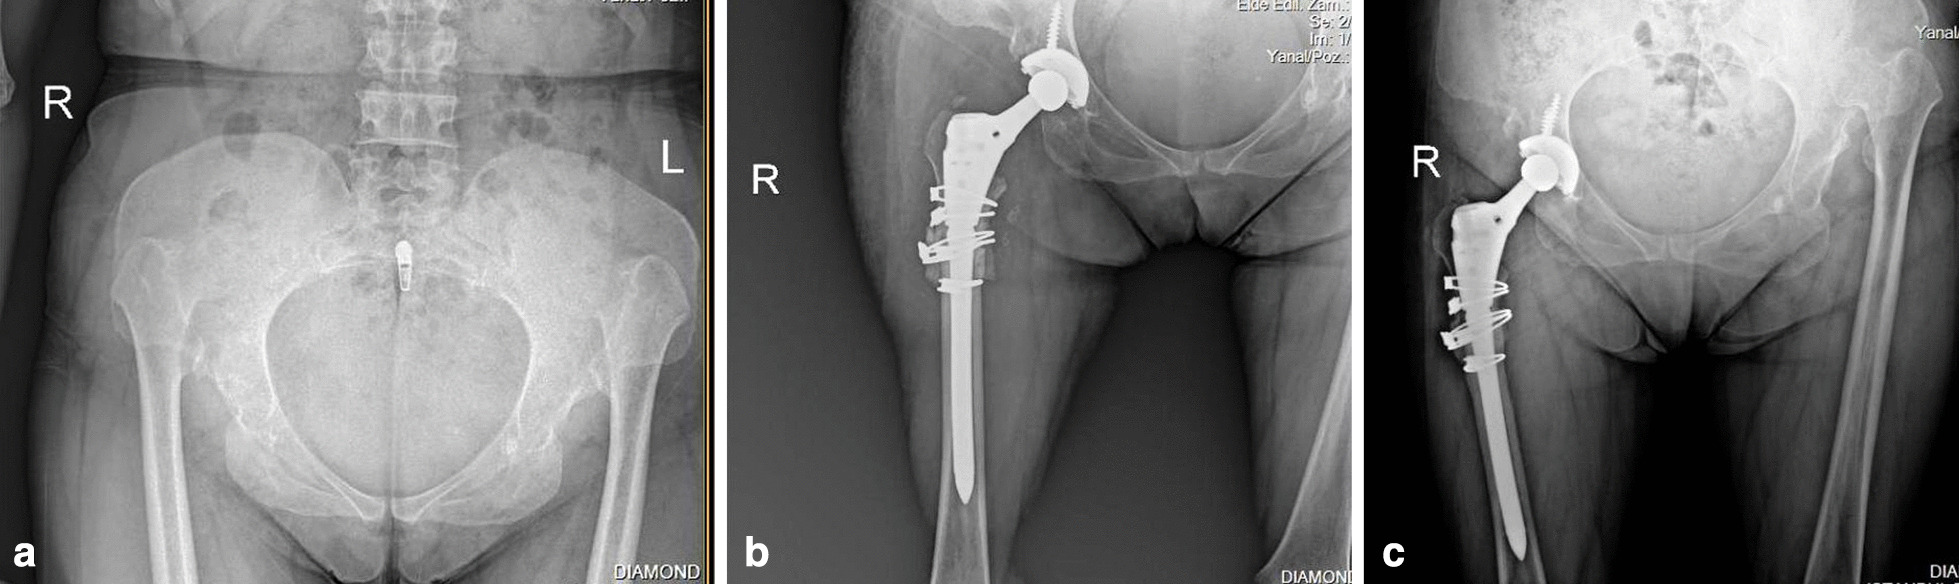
Radiographs of a 48 years old female with high hip dislocation. a Preoperative anteroposterior view. b Early postoperative radiographic image. Hip was reconstructed at the level of the anatomic hip center by cementless modular (Arcos, Zimmer, Biomet) total hip arthroplasty combined with transverse osteotomy and the osteotomy site was wrapped with the osteotomy fragments, stabilized using cerclage wires. c Postoperative radiographic image at 3 years follow-up, bone union was detected at the osteotomy site and no radiolucent lines around the femoral stem were found
